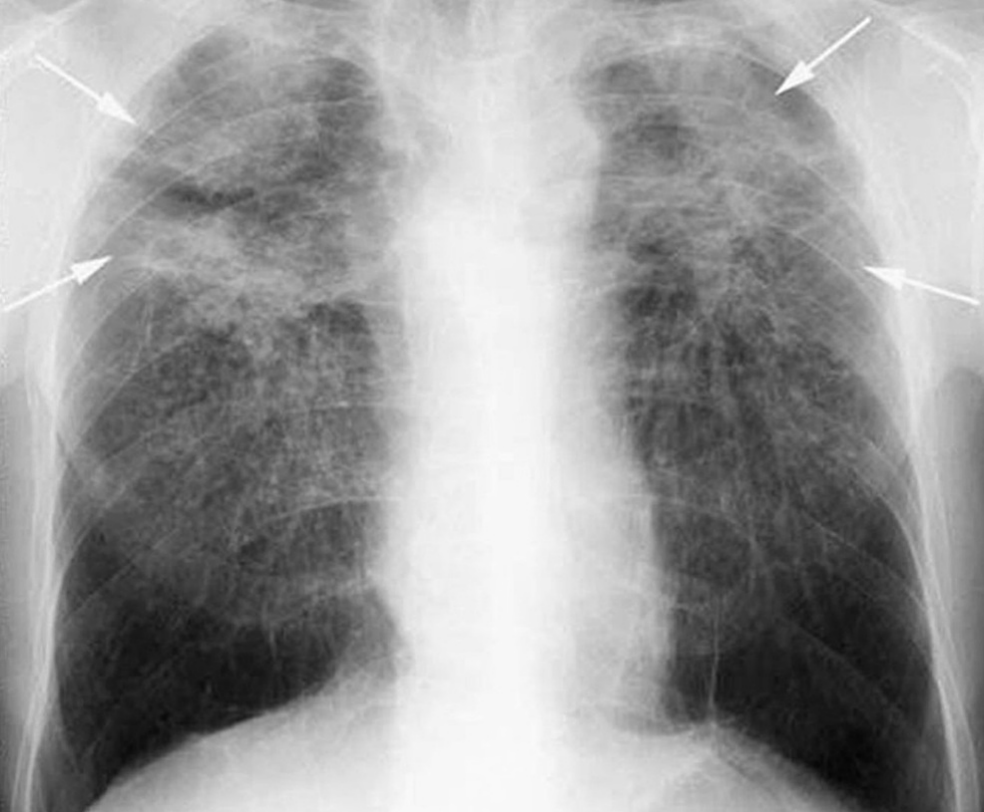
Chest x-ray upon admission: PA view, arrows showing bilateral diffuse infiltrates. Cardiomegaly is also noticed, later confirmed on CT and echocardiography. PA: posteroanterior
